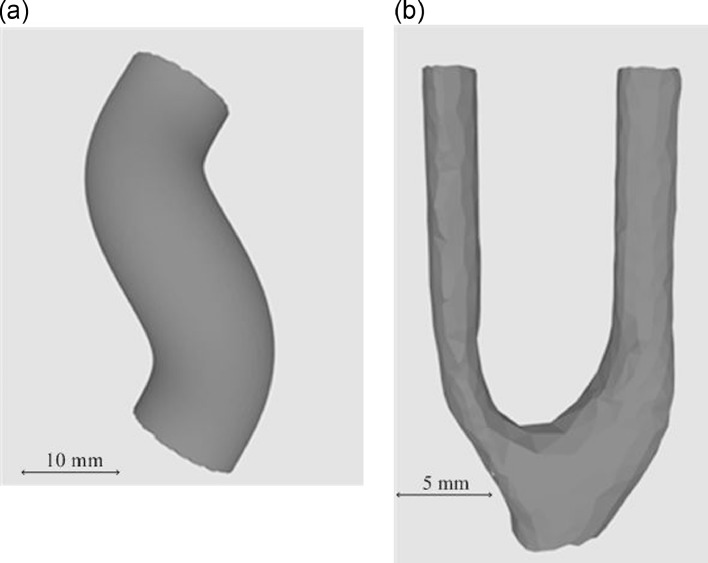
Geometries of cases 2 and 3: (a) helix; (b) carotid bifurcation (surface geometry from ultrasound scan).
